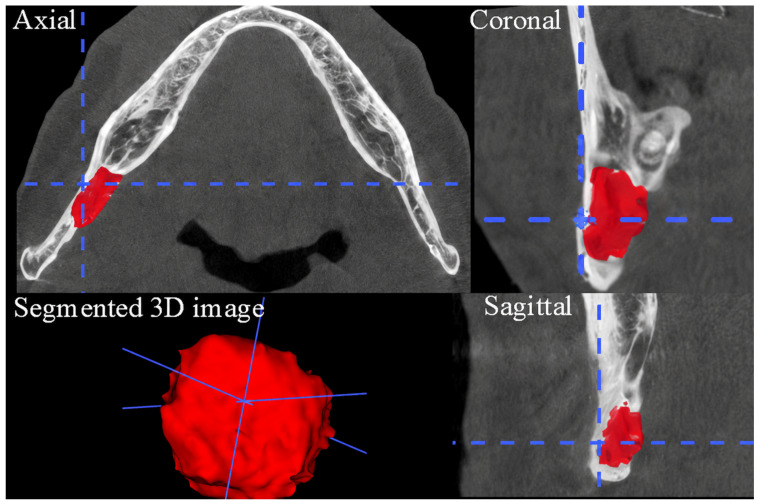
Three-dimensional visualization of SBC and volumetric dimension measurement. Axial, coronal, and sagittal CBCT images demonstrate the anatomical location of the lesion. The segmented area is shown in red, representing the SBC volume obtained through semi-automatic segmentation. Blue dashed lines indicate the crosshair reference axes used for spatial localization of the lesion across orthogonal planes.
